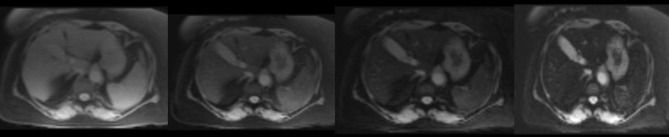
MRI 1.5 T (Siemens Avanto), using multi-TE gradient echo T2* MRI technique (using Garbowski method). Liver intensity is normal seen with the longest TE (14.68 msec). T2* =7.4 ms, corresponding to < 5 mg Iron/ g liver dry weight.
